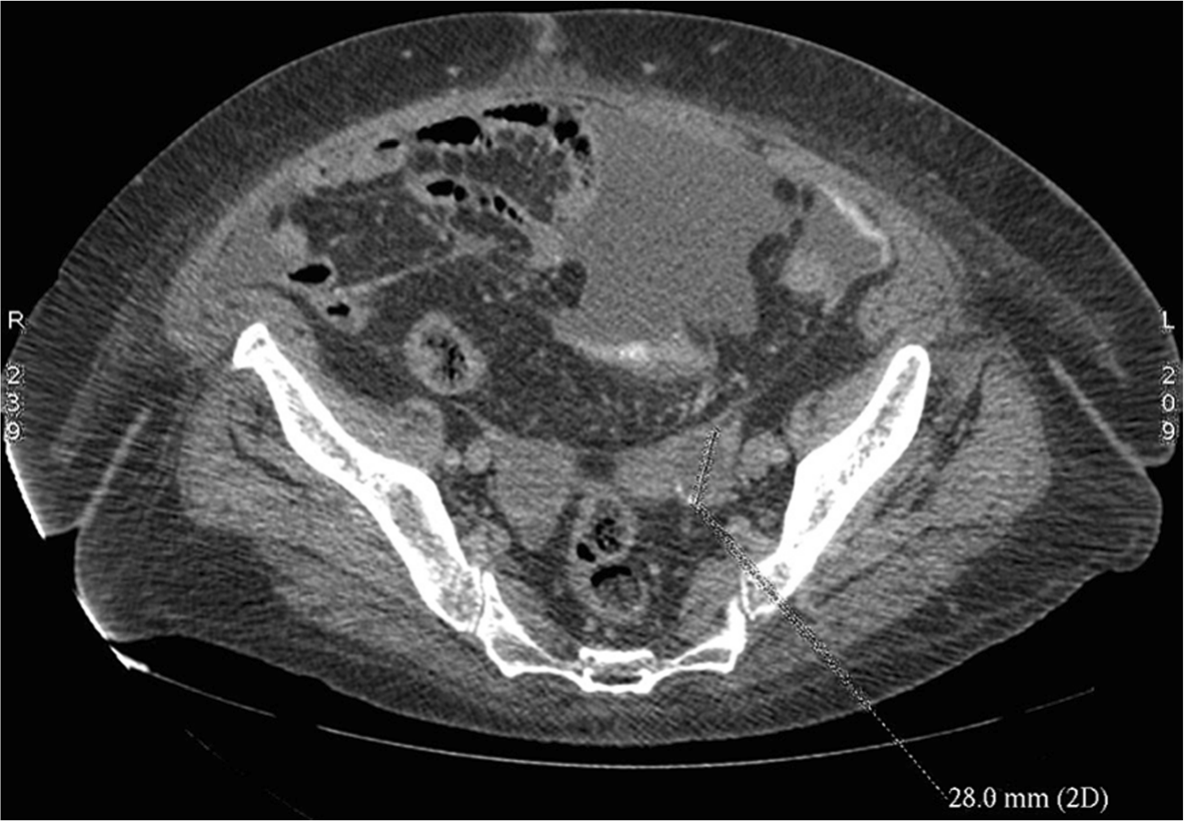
Axial computed tomography image showing an exophytic lesion of the right kidney.
